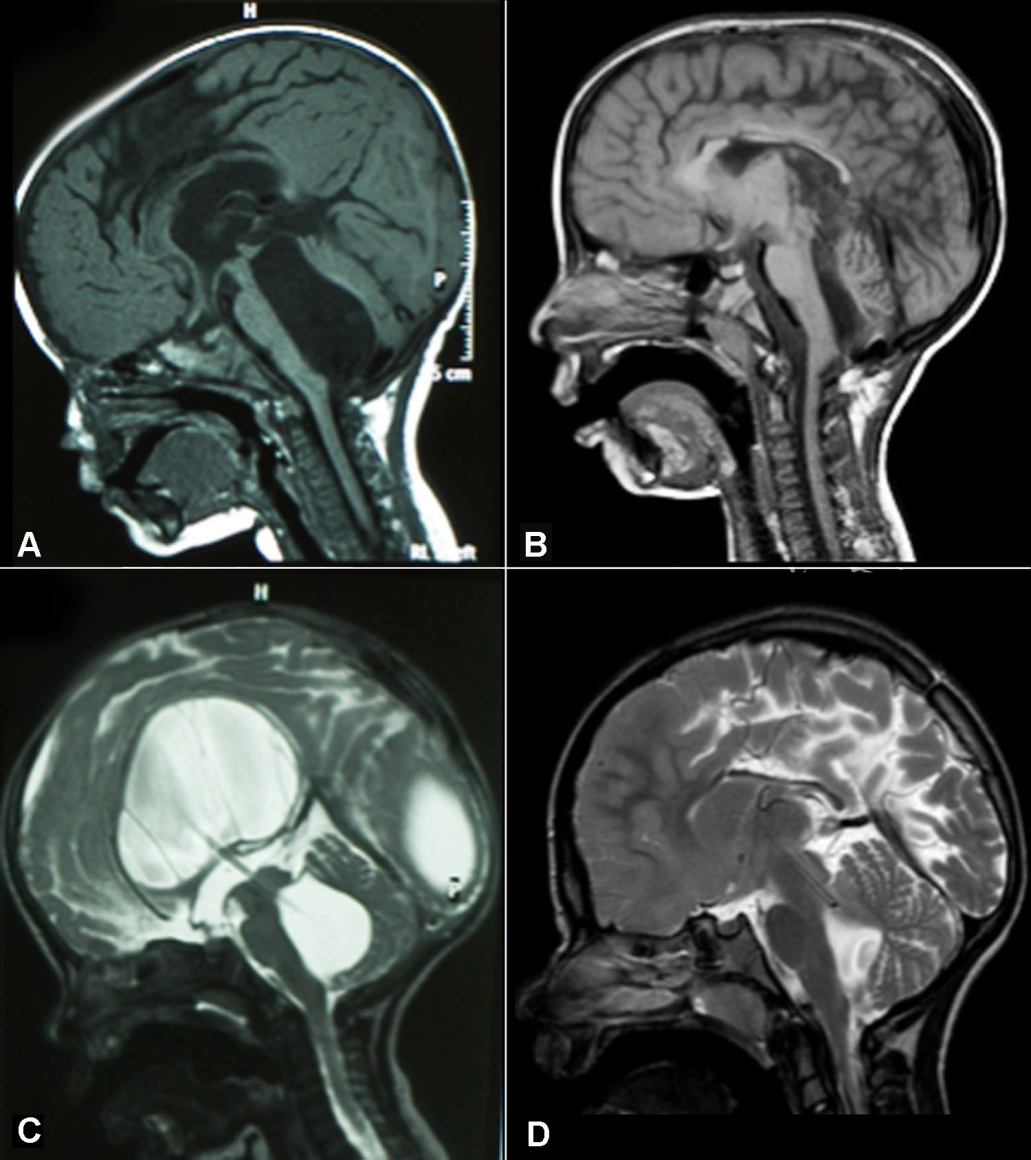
Preoperative (A) and postoperative (B) T1-weighted MRI sequences of a patient suffering from isolated fourth ventricle that underwent and endoscopic aqueductoplasty, no stent was left in the sylvian aqueduct. Preoperative (C) and postoperative (D) T2-weighted MRI sequences of a child presenting with isolated fourth ventricle that underwent and endoscopic aqueductoplasty. In this patient, a stent was placed across sylvian aqueduct
